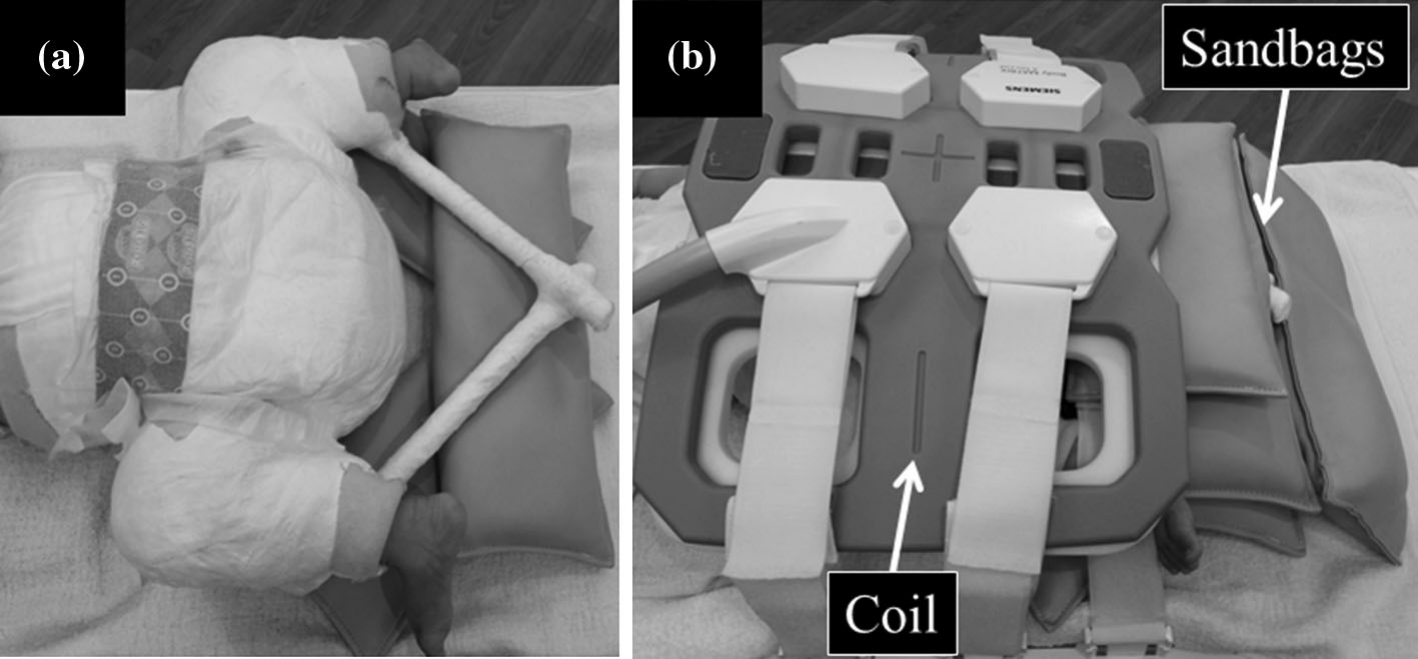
Photographs of the system used to immobilize patient for magnetic resonance imaging (MRI), before (a) and after immobilization (b). Infants were placed on the examination table and were immobilized using MRI dedicated sandbags and coil
